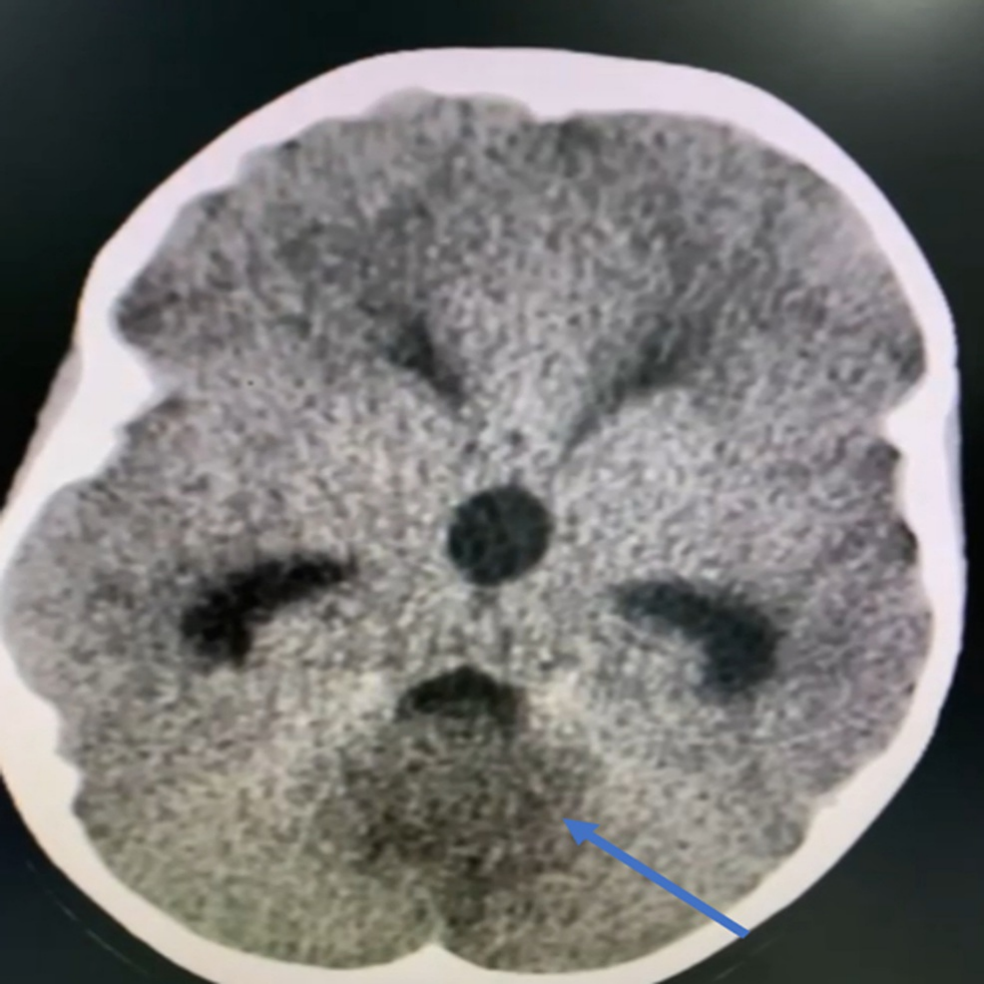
Preoperative image - CT brain axial cut shows obstructive hydrocephalus showing posterior fossa lesion
